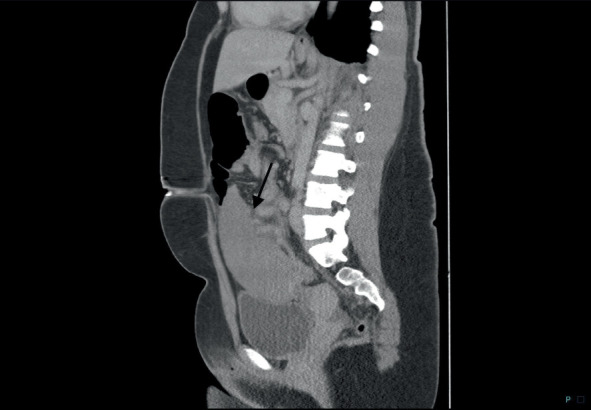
CT image shows an anteriorly located spleen in the lower quadrants of the abdomen indicated by the arrow.
